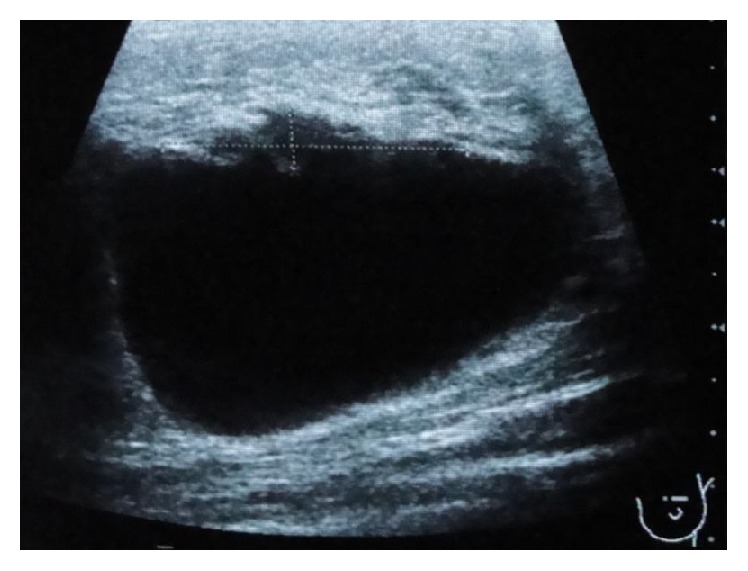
Ultrasound of the left breast (2nd evaluation): cyst measuring 44 mm with irregular and hypoechogenic vegetation of 6 × 27 mm.
